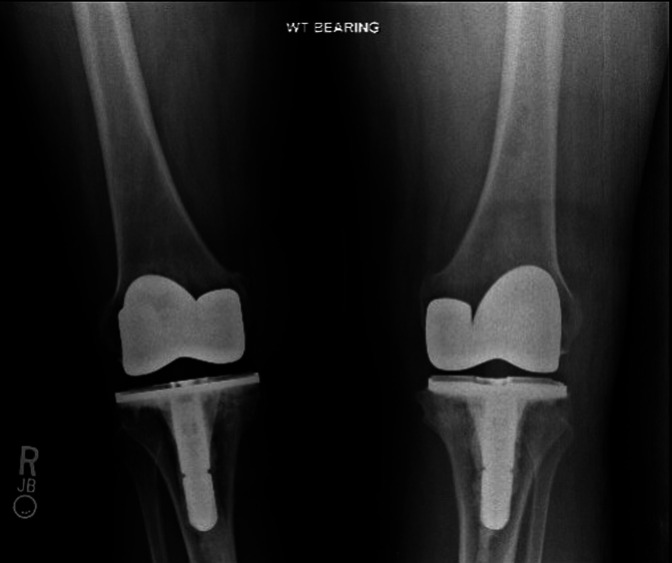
Postoperative anterior-posterior weight-bearing plain radiograph demonstrating bilateral total knee arthroplasty in good position and alignment.
